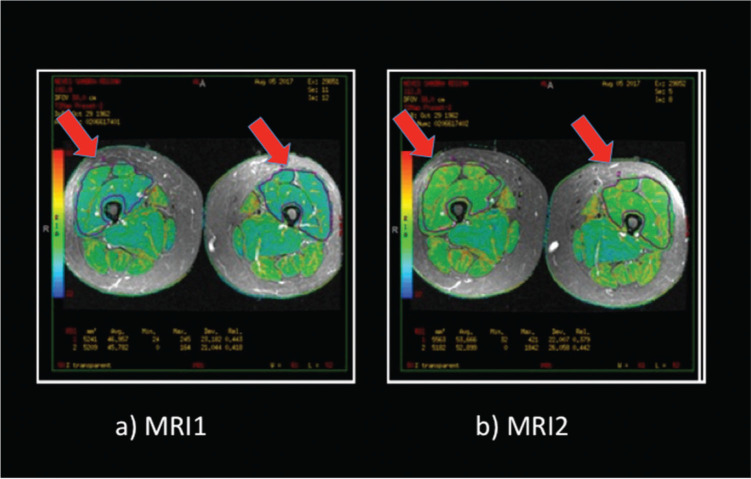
Nuclear magnetic resonance imaging (a) in preexercise period (MRI1) and (b) in postexercise period (fatigue) of the right and left thighs (MRI2). Indicated area corresponds to the quadriceps muscle.
